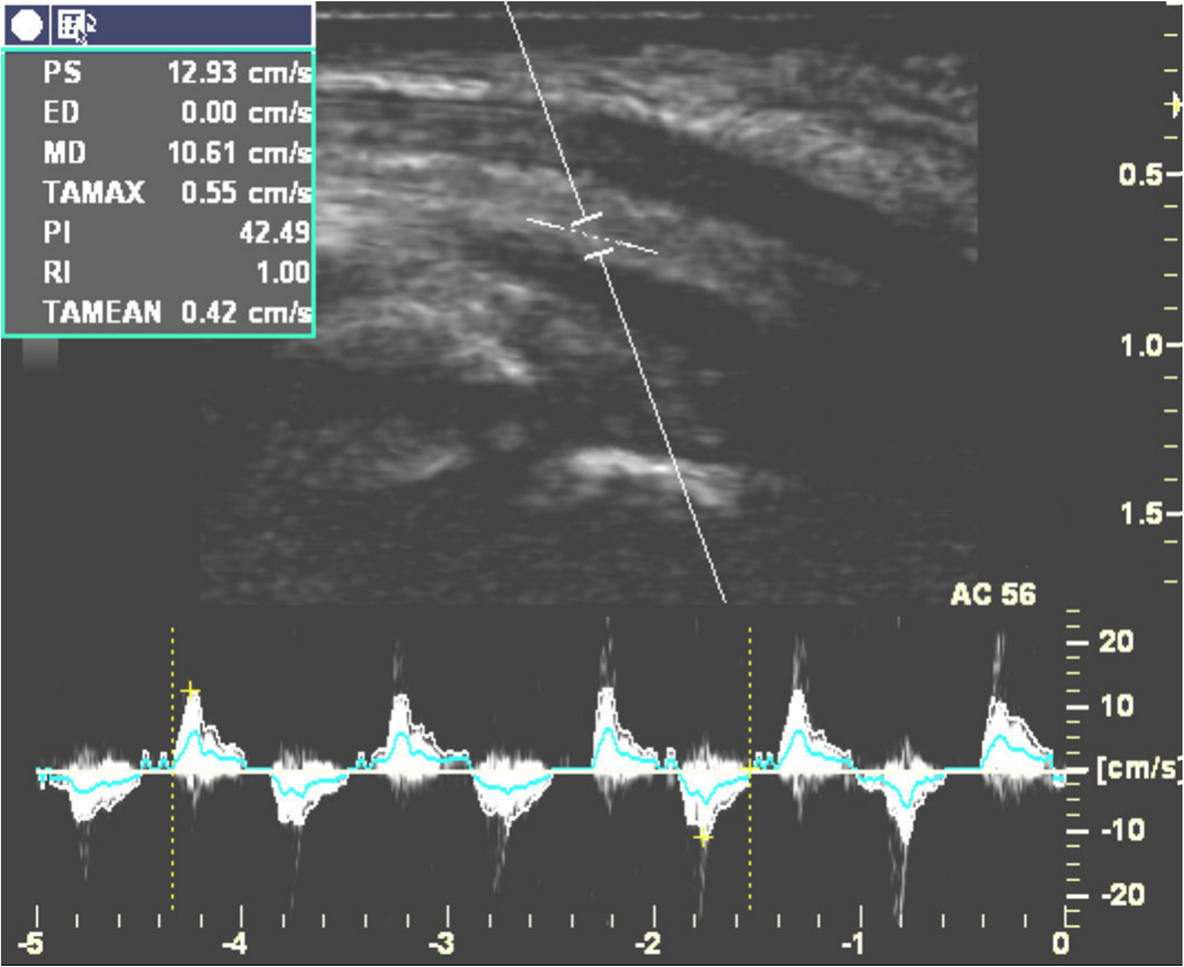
Doppler waveforms for tendon excursion of flexor digitorum superficialis when subjects performed repetitive flexion/extension of index finger at a speed once per second
